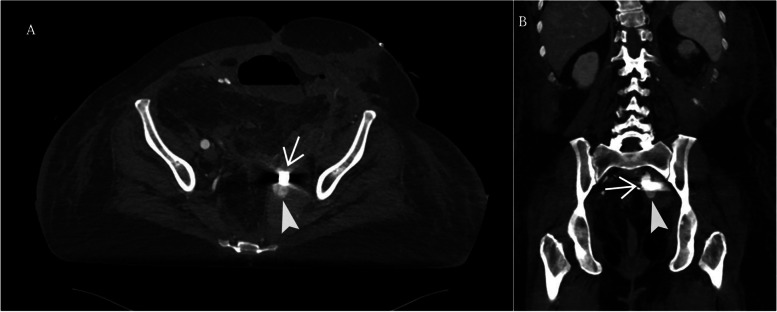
CT angiogram of the left internal iliac artery 7 days after embolization of mycotic pseudoaneurysm with onyx A) Axial and B) MPR coronal CT angiogram demonstrates enlarged left internal iliac artery mycotic pseudoaneurysm sac measuring 8 × 4 cm with partial filling of the sac (arrowhead) and prior onyx(arrow)
